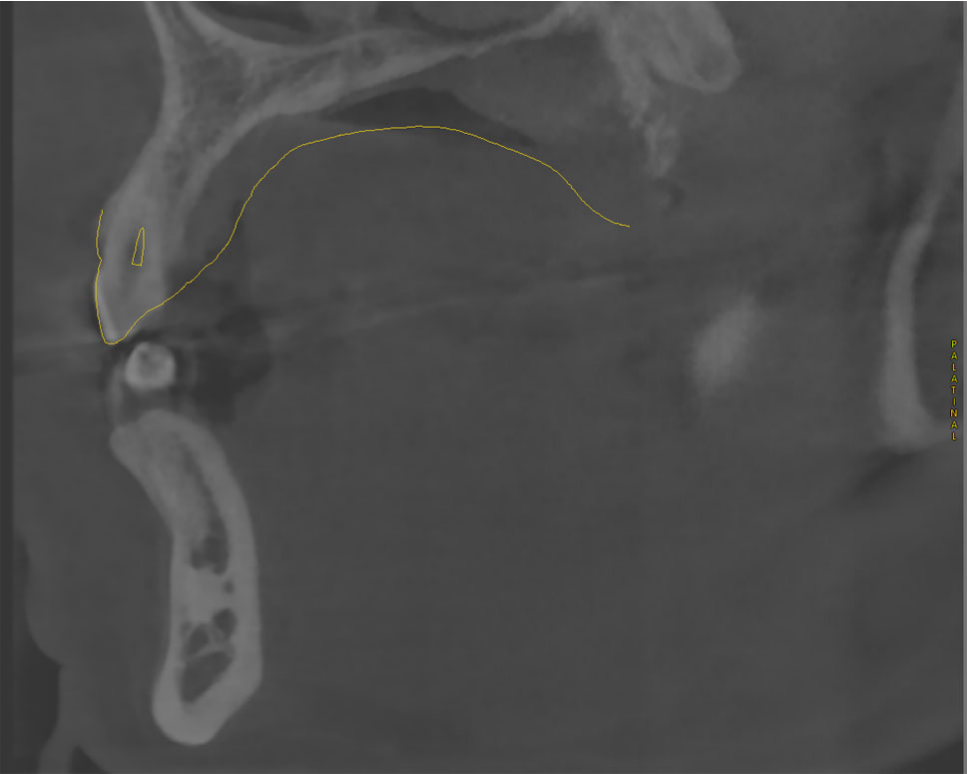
Paramedian cut of the CBCT-scan superimposed with a dental wax-up and the SSM-based tooth axis reconstruction. The predicted tooth axis of tooth 23 (yellow) is located at a similar position as the actual root canal of the tooth 23. The comparison of calculated tooth axis with dental roots of existent teeth enables to presume the validity of the SSM-based prediction of missing roots
